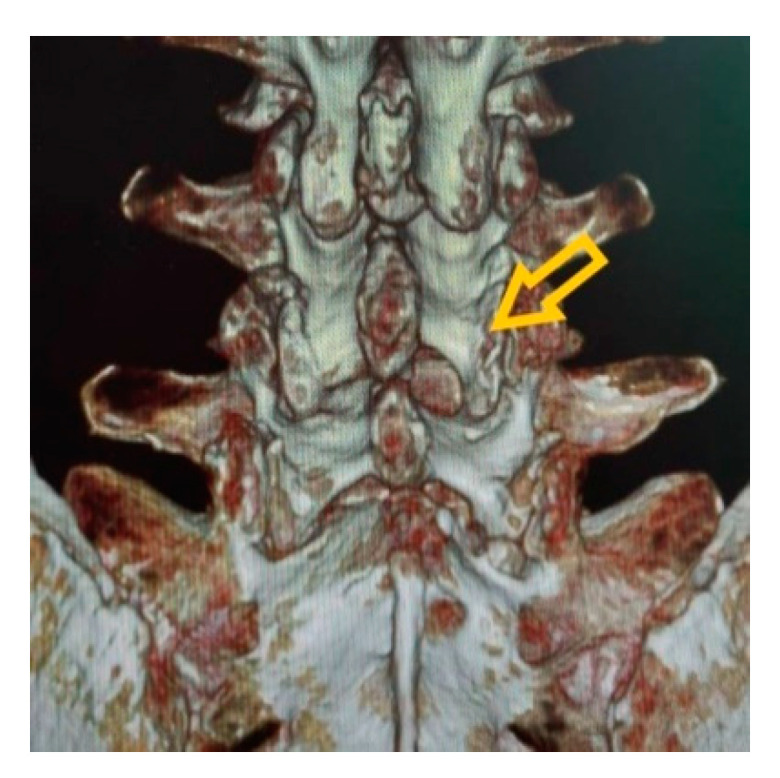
Postoperative CT scan after interlaminar percutaneous endoscopic decompression (PED). Yellow arrow indicates area of laminotomy.
